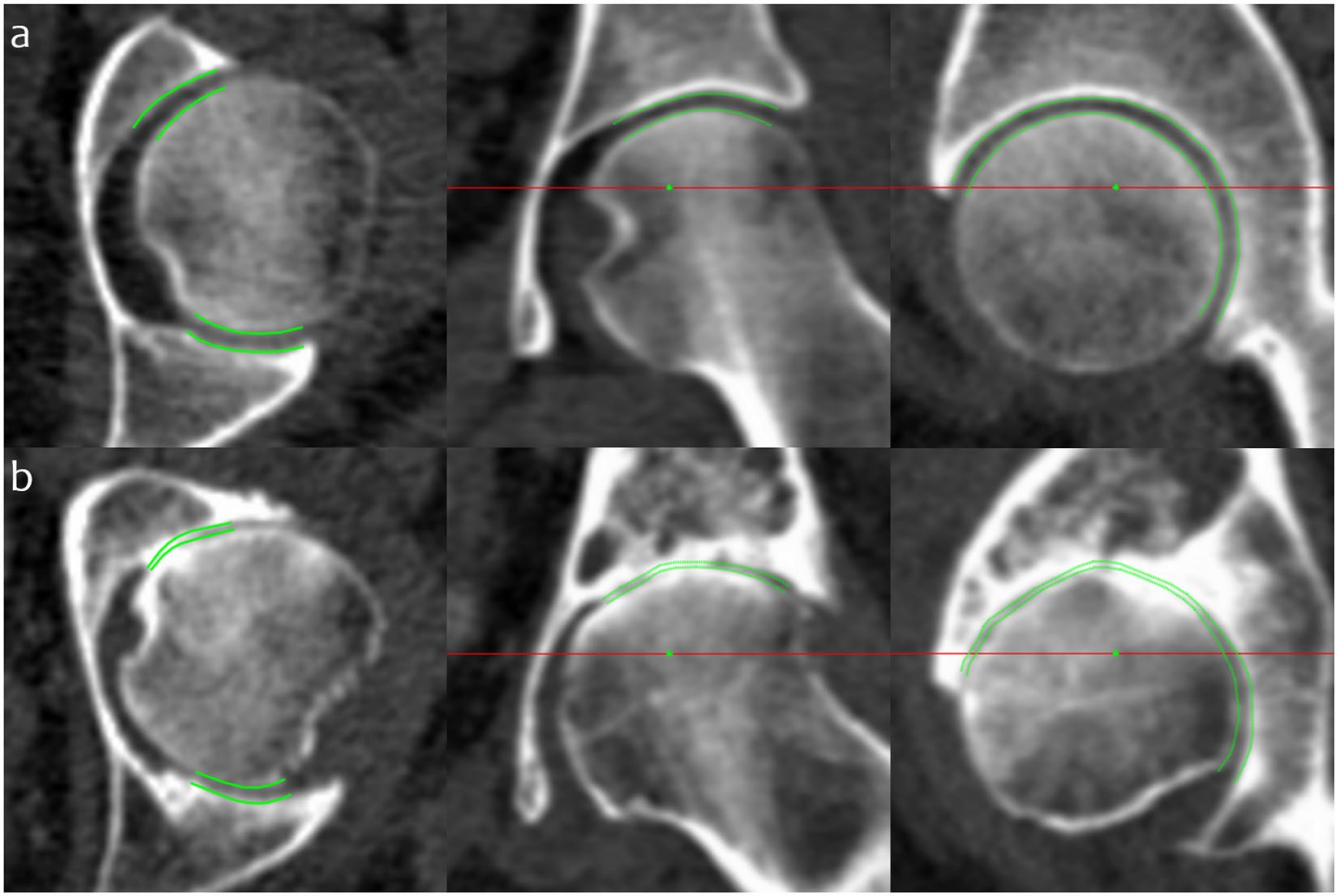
JSM performance at the extremes of disease. Joint space margins are shown in green as seen in axial, coronal and sagittal planes (left to right) at two left hips. (a) An individual with a radiographic K&L grade of 0 (no disease) and minimum joint space width of 2.0 mm. (b) An individual with a radiographic K&L grade of 3 (moderate disease) and minimum joint space width of 0 mm. This demonstrates that JSM is still able to represent joint space width as it approaches zero, important for analysis in advanced disease.
